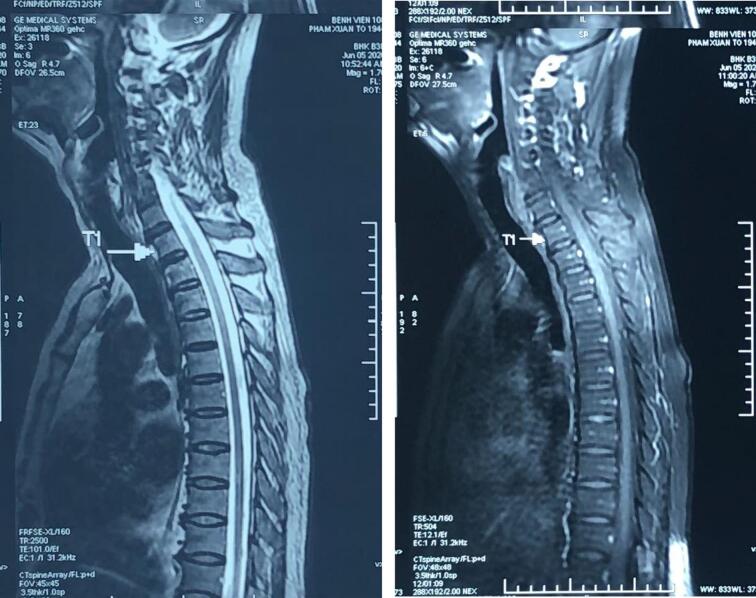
At the T1-T12 level, MRI showed increased intramedullary signal intensity.
